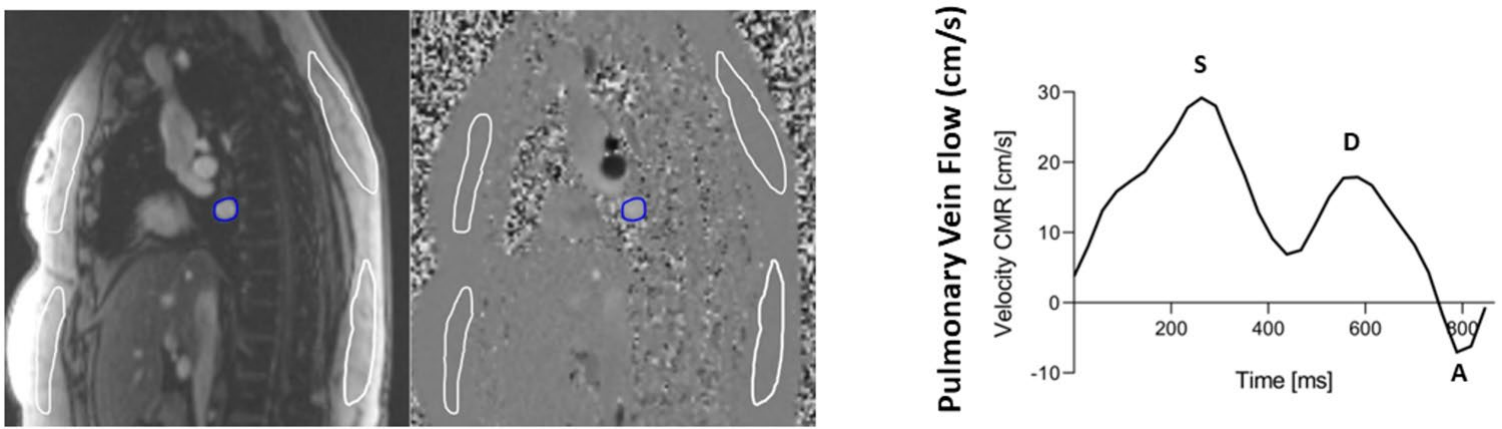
Magnitude and phase images from magnetic resonance imaging examination and the corresponding flow curves in the pulmonary vein in a patient with pulmonary arterial hypertension. Time velocity curves of the right lower pulmonary vein (blue) showing systolic (S), Diastolic (D) and the atrial reversal (A) flows.
